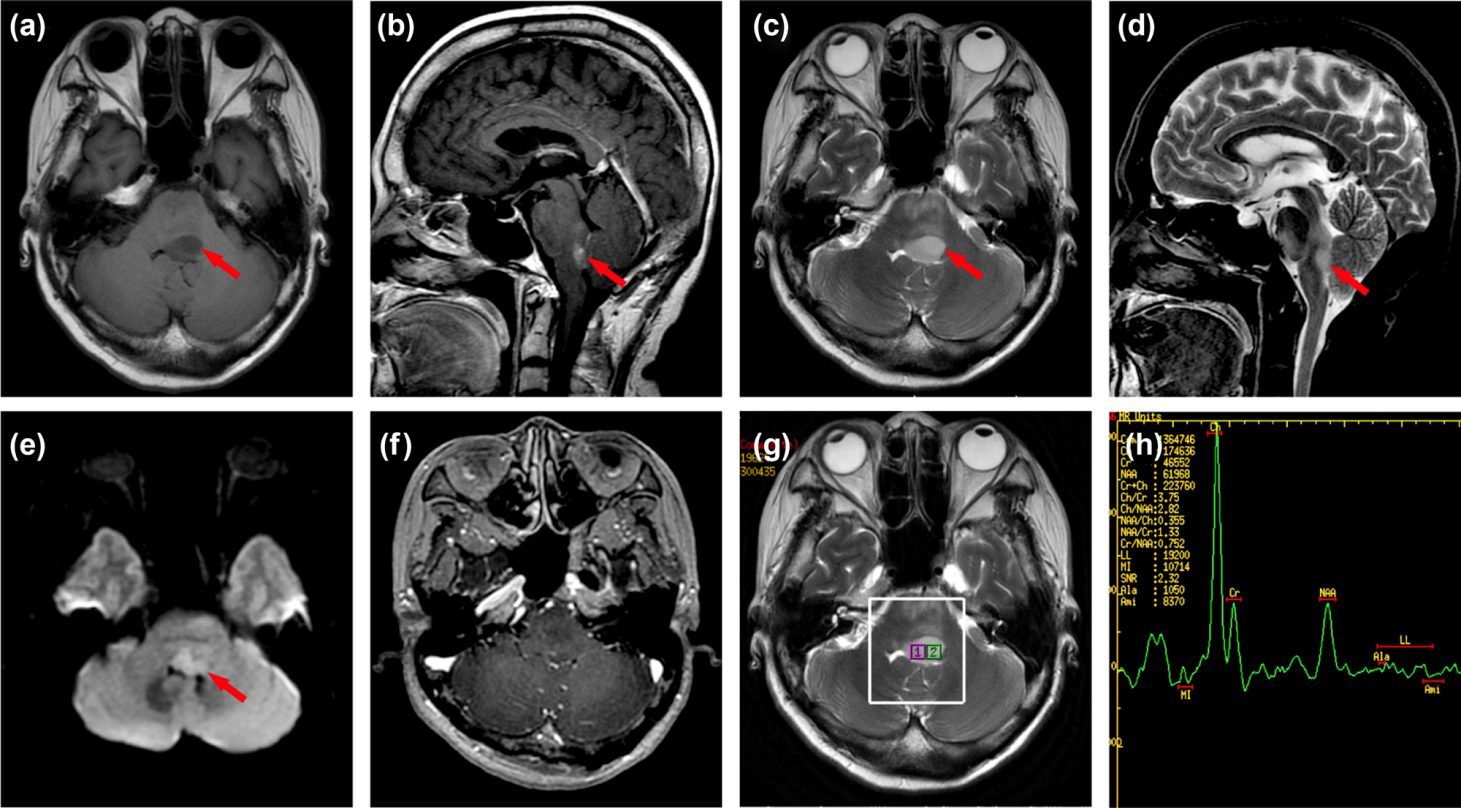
The patient’s first cranial MRI results show that the brainstem is enlarged and swollen. (a) T1WI revealed a mass of abnormal low signal intensity in the brainstem near the fourth ventricle (arrow). (b) T1-FLAIR, (c) T2WI, (d) T2-FLAIR, and (e) DWI showed that the abnormal mass in the brainstem had high signal intensity (arrows). (f) There was no obvious enhancement of the abnormal signal. (g and h) Cho/NAA = 2.83, Cho/Cr = 3.75, NAA/Cr = 1.33 of MRS point of interest 2.
